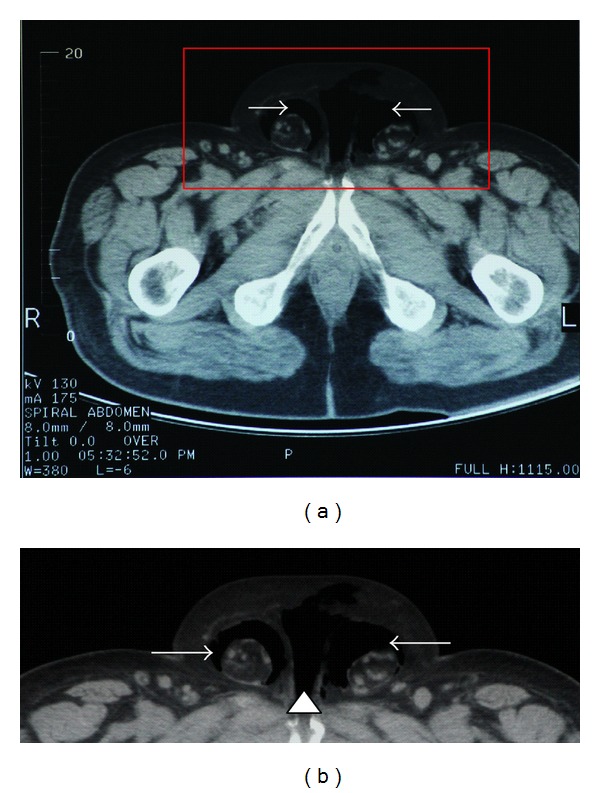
(a) Computed tomography scan of the pelvis. Air (white arrows) is visualized within the scrotal sac (indicative of pneumoscrotum). (b) Magnification of the red frame of (a). Air appears to the scrotum (white arrows) and at the base of the penis (head arrow).
